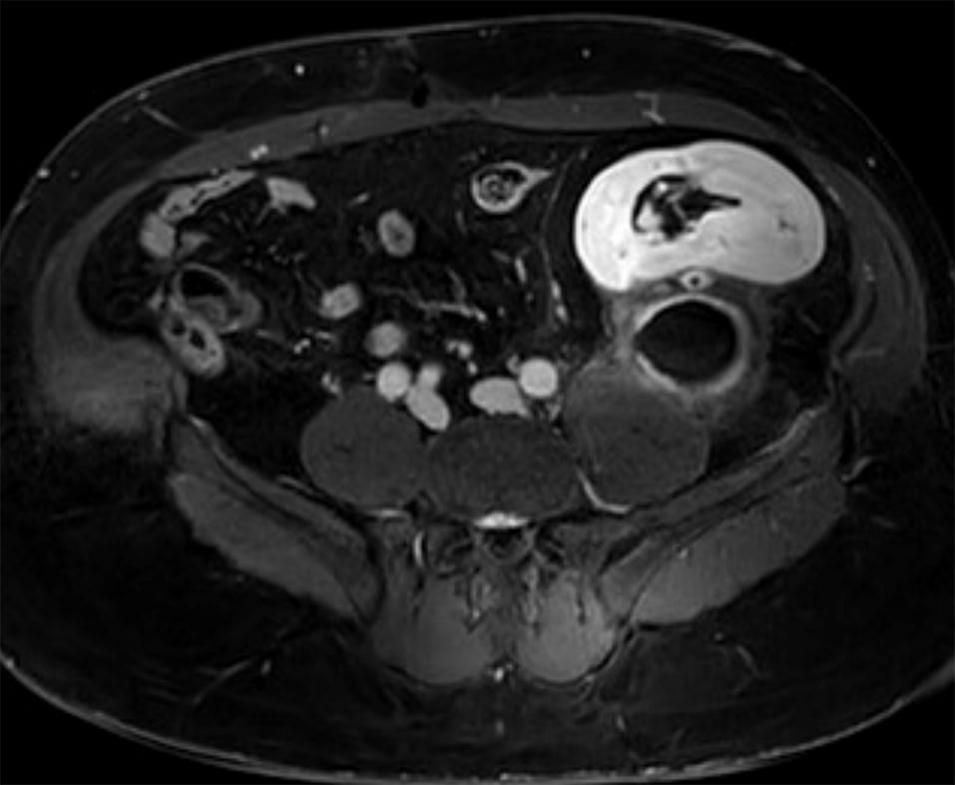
MRI showing the fluid collection with a thick and heterogeneous capsule before the second aspiration and drainage. The view is similar to Fig. 3, allowing comparison
